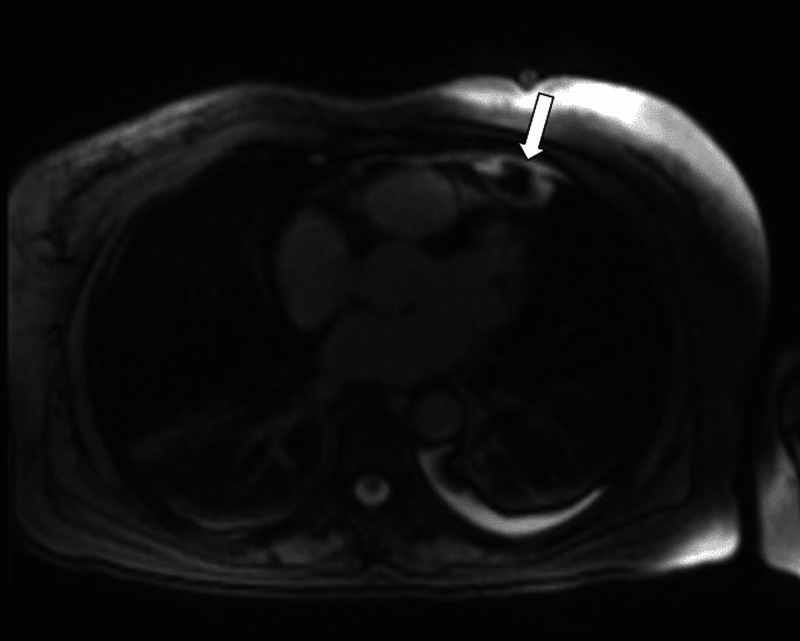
Magnetic resonance image T1-weighted high-intensity signal showing increased enhancement in the rim suggestive of fat necrosis as indicated by an arrow. Image provided by the Beaumont Hospital, Royal Oak with permission for use.
